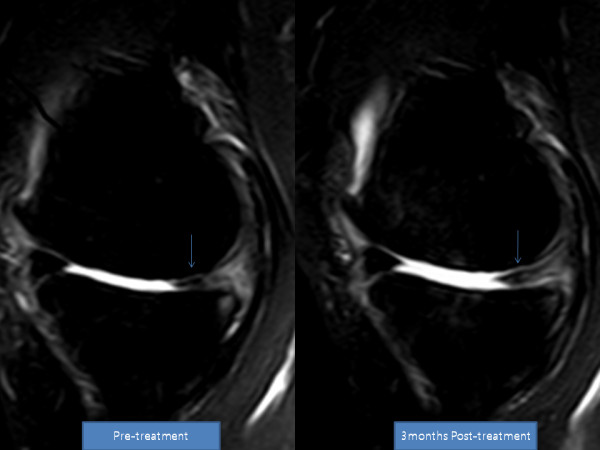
MRI sagittal T2 view of the knee. Pre-treatment and post-treatment MRI shows increased height of medial meniscus cartilage and articular cartilage (arrow).
